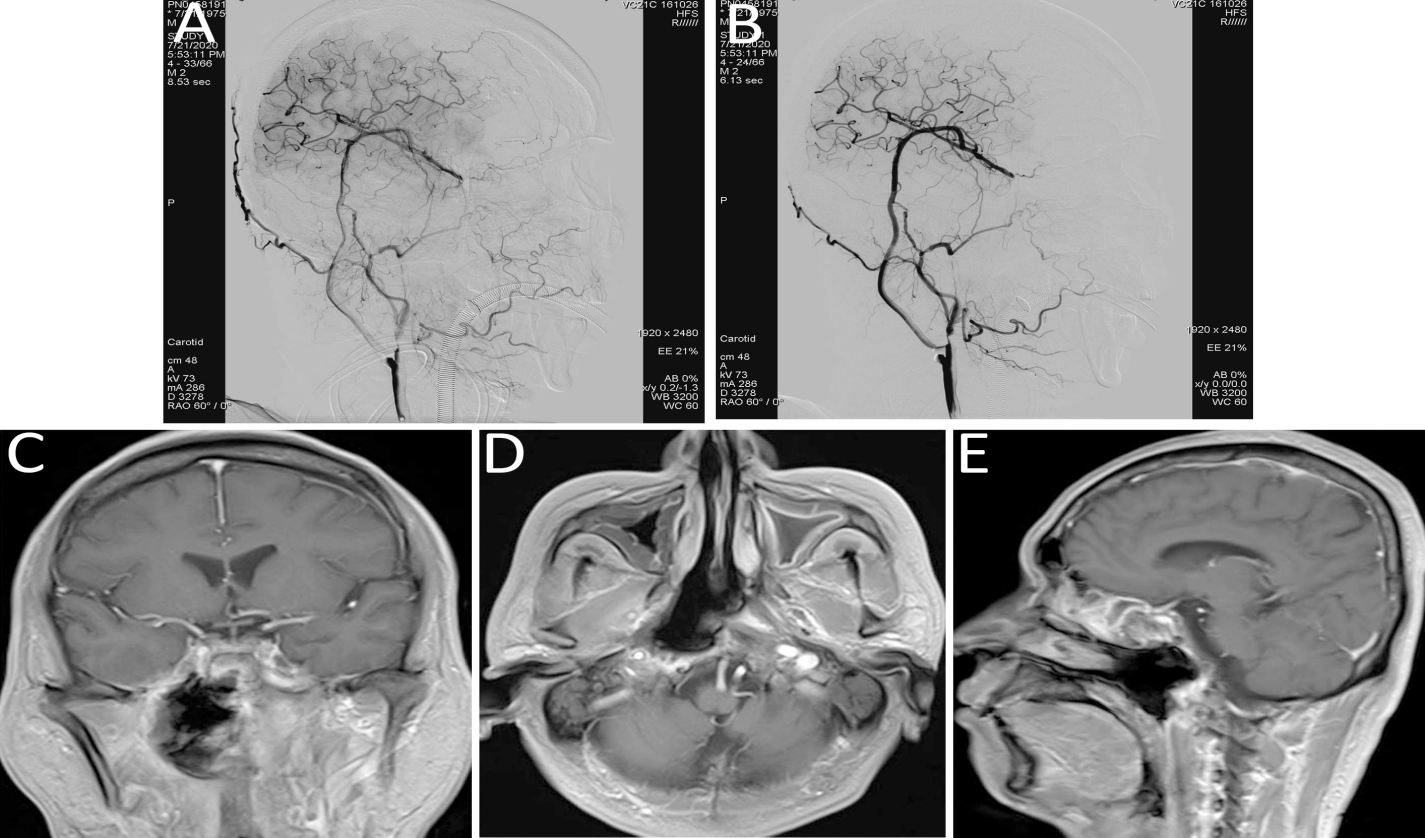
(A, B) Immediate post-bypass angiography confirmed patency of the bypass graft, with sufficient perfusion of the right middle cerebral artery and retrograde supply to the ophthalmic artery. No perfusion was observed in the right internal carotid artery at the cervical (C1), petrous (C2), and cavernous (C4) segments. (C–E) Follow-up head MRI demonstrated significant tumor shrinkage and satisfactory local disease control.
